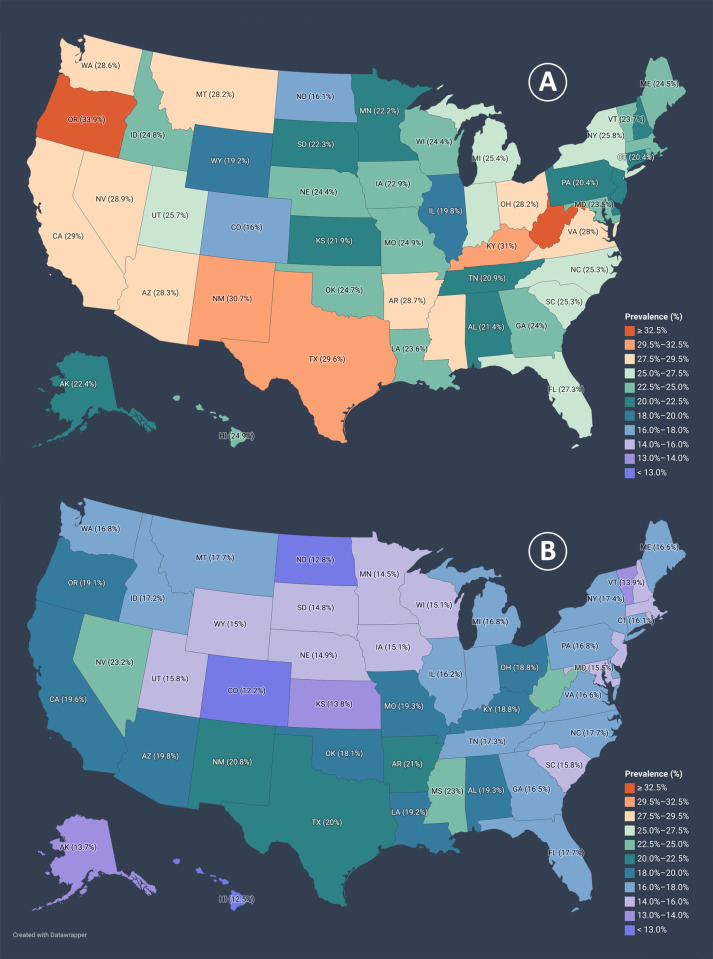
State-wise Prevalence of Unfavorable Self-reported General Health among COVID Survivors. (A) Shows the state-wise prevalence of unfavorable SRGH among COVID survivors with PCCs. (B) Shows the state-wise prevalence of SRGH among all COVID survivors. AL: Alabama, AK:Alaska, AZ: Arizona, AR: Arkansas, CA: California, CO: Colorado, CT: Connecticut, DE: Delaware, DC: District of Columbia, FL: Florida, GA: Georgia, HI: Hawaii, ID: Idaho, IL: Illinois, IN: Indiana, IA: Iowa, KS: Kansas, KY: Kentucky, LA: Louisiana, ME: Maine, MD: Maryland, MA: Massachusetts, MI: Michigan, MN: Minnesota, MS: Mississippi, MO: Missouri, MT: Montana, NE: Nebraska, NV: Nevada, NH: New Hampshire, NJ: New Jersey, NM: New Mexico, NY: New York, NC: North Carolina, ND: North Dakota, OH: Ohio, OK: Oklahoma, OR: Oregon, PA: Pennsylvania, RI: Rhode Island, SC: South Carolina, SD: South Dakota, TN: Tennessee, TX: Texas. UT: Utah. VT: Vermont, VA: Virginia, WA: Washington, WV: West Virginia, WI: Wisconsin, WY: Wyoming.
